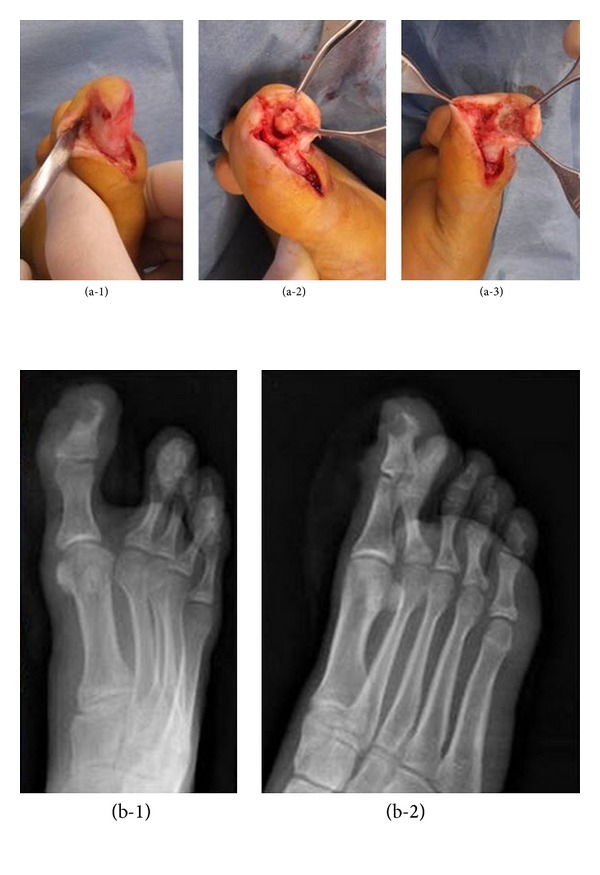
(a-1) Longitudinal incision at medial side of the distal phalanx of the toe. (a-2) Dissection of the osteoid osteoma. (a-3) Curettage of the osteoid osteoma. (b-1, b-2) Postoperative radiographies.
